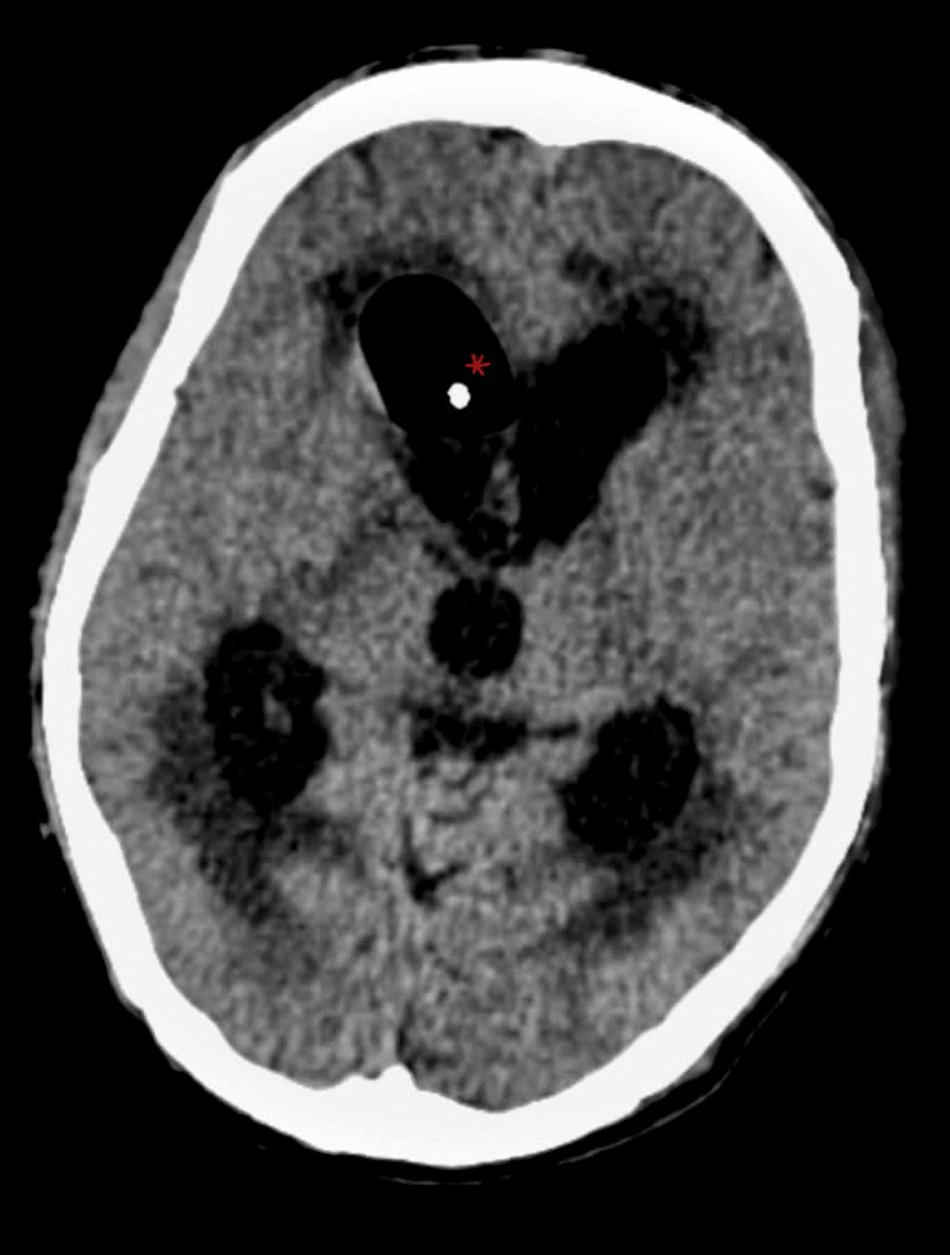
CT Brain image showing dilated bilateral lateral ventricles with periventricular ooze and Ommaya shunt tip in frontal horn of right lateral ventricle.
